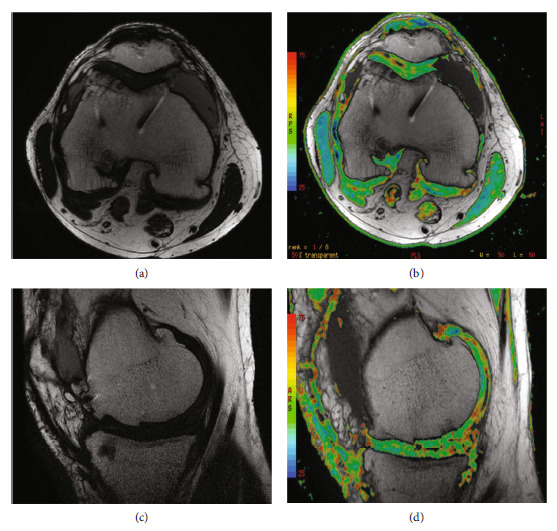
Four-year postoperative MRI. (a) Axial metal artifact reduction sequence (MARS) image demonstrates previous trochlear osteochondral allograft well integrated with intact articular cartilage. (b) T2 mapping axial image with maintained patellofemoral articular cartilage. (c) Sagittal MARS image with well-incorporated medial femoral allograft and preserved articular continuity. (d) Sagittal T2 mapping image demonstrating intact articular cartilage surface at medial femoral condyle allograft.
